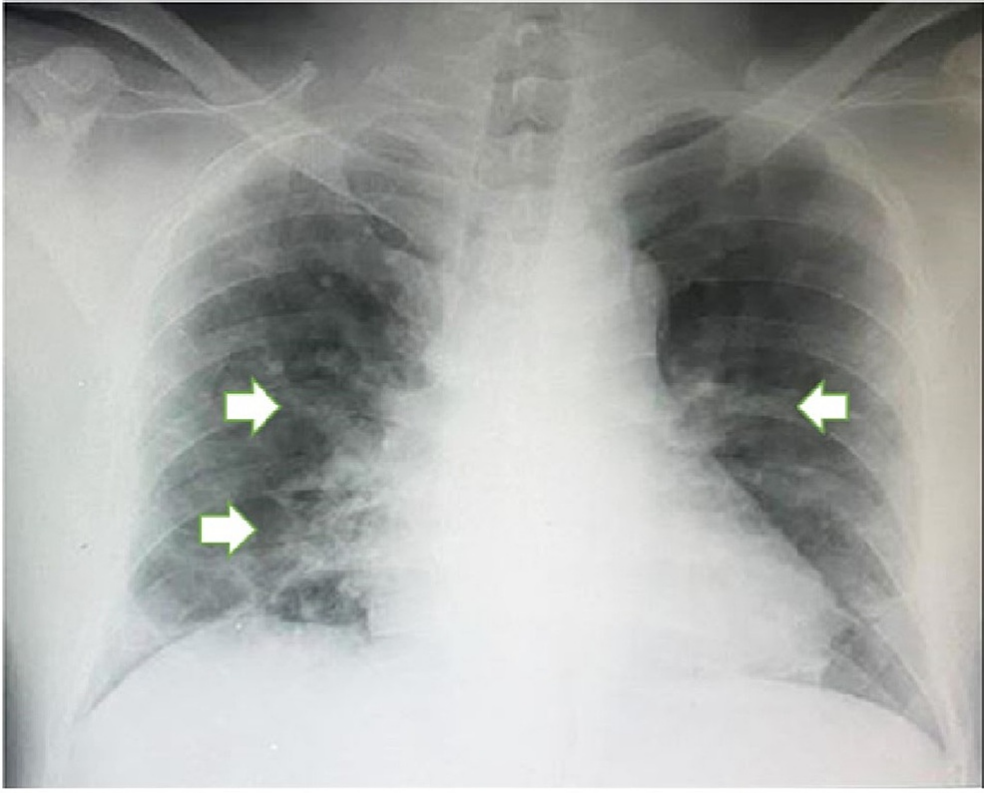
Anterior-posterior chest radiograph of the patient with ground glass opacity in both middle and lower zones (white arrows) with preservation of lung markings.
